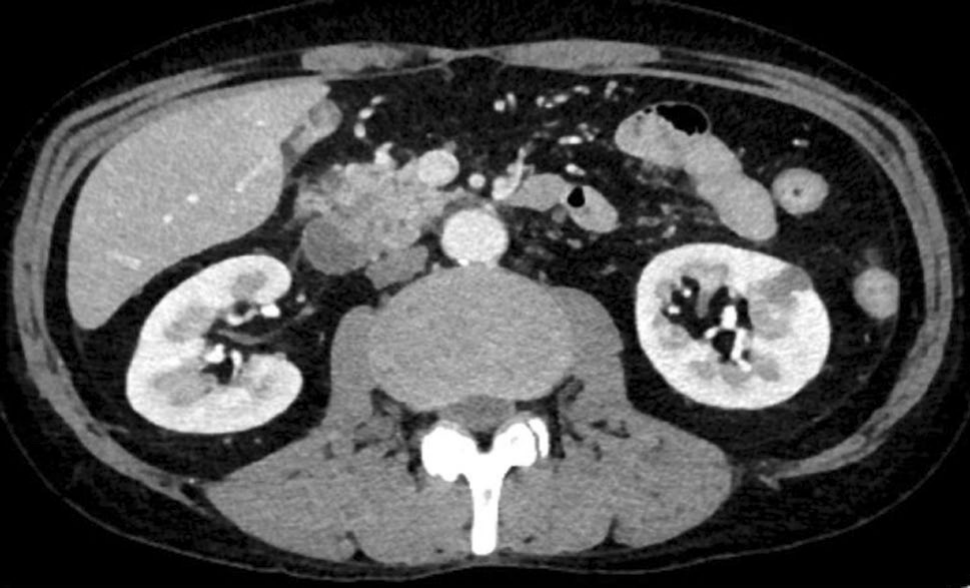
After six courses of R-CHOP therapy, a 95.3% lesion rate of reduction was confirmed on CT, which was judged as a partial response
